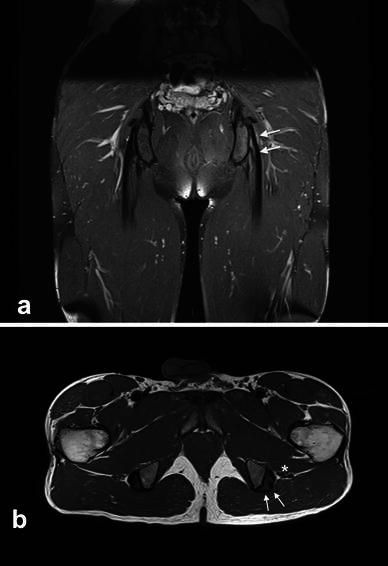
Magnetic resonance images of a 27 years old male hurdler with chronic posterior left sub-gluteal pain. a Proton density-weighted coronal image showing tendinosis of proximal left semimembranosus tendon (*arrows*). b T1-weighted axial image clear defines tendinosis and not tear (*arrow*). Left sciatic nerve runs immediately lateral to the tendon (*asterisk*)
